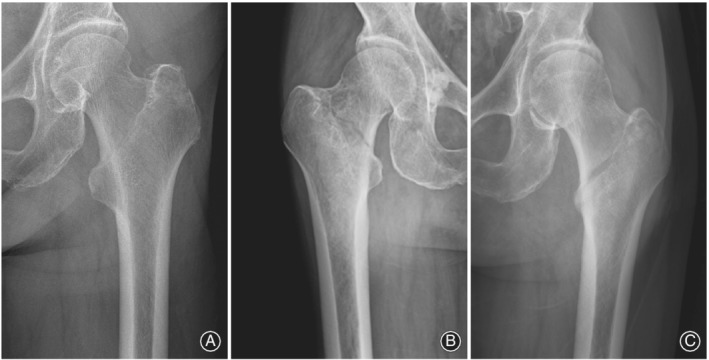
Radiograph of a typical basicervical intertrochanteric fracture: (A) Shanghai Punan Hospital, (B) Shanghai General Hospital, and (C) Shanghai Seventh People's Hospital.
